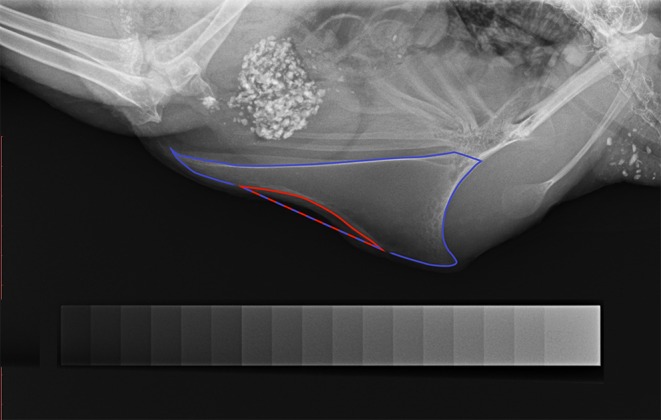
Radiograph of a deviated keel bone. The keel bone surface area is circumscribed with blue color; the area of deviation is circumscribed with red color. The blue-red line marks the straight line between both extremes of the deviated outline. The aluminum step-wedge can be seen at the bottom of the figure.
